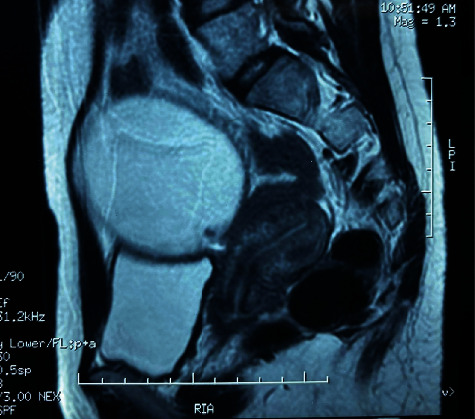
Magnetic resonance imaging showing abdominopelvic mass.
